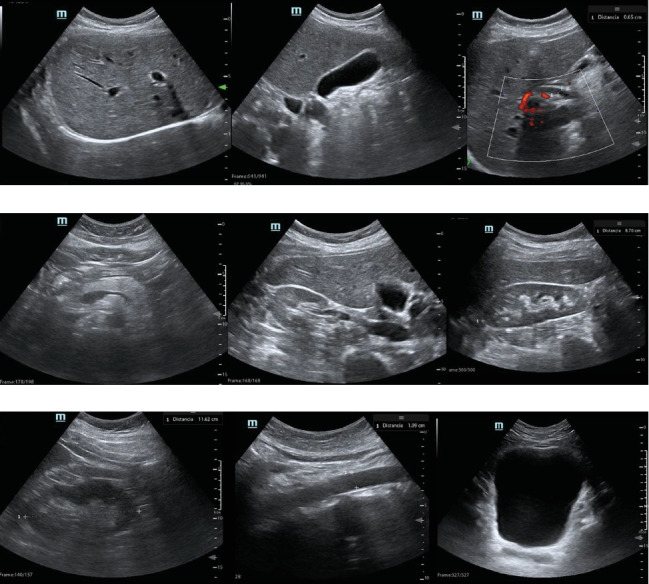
Examples of anatomical structures visualized on telerobotic ultrasound. (a) (left to right) liver, gallbladder, and main bile duct. (b) Pancreas and right kidney (axial and longitudinal views). (c) left kidney, aorta, and urinary bladder.
